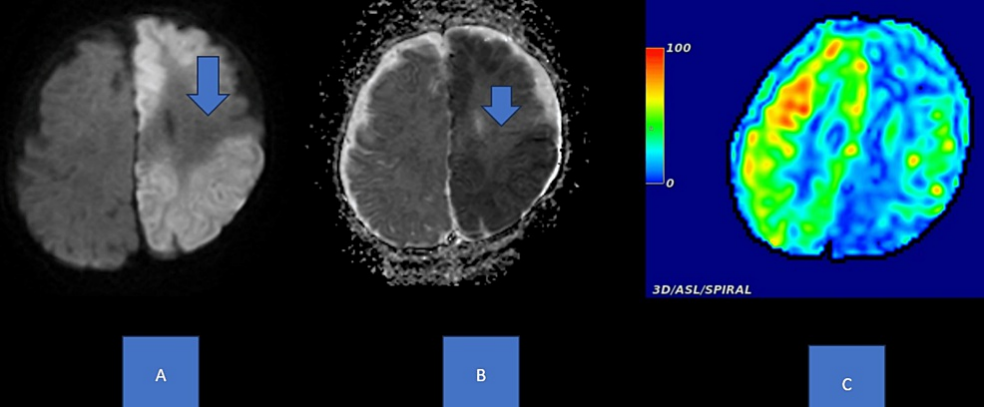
Axial DWI, ADC, and ASL demonstrate a large area of restricted diffusion and decreased cerebral blood flow in the left cerebral hemisphere with some sparing of the left frontal lobe. DWI: diffusion-weighted imaging; ADC: apparent diffusion coefficient; ASL: arterial spin labeling.
